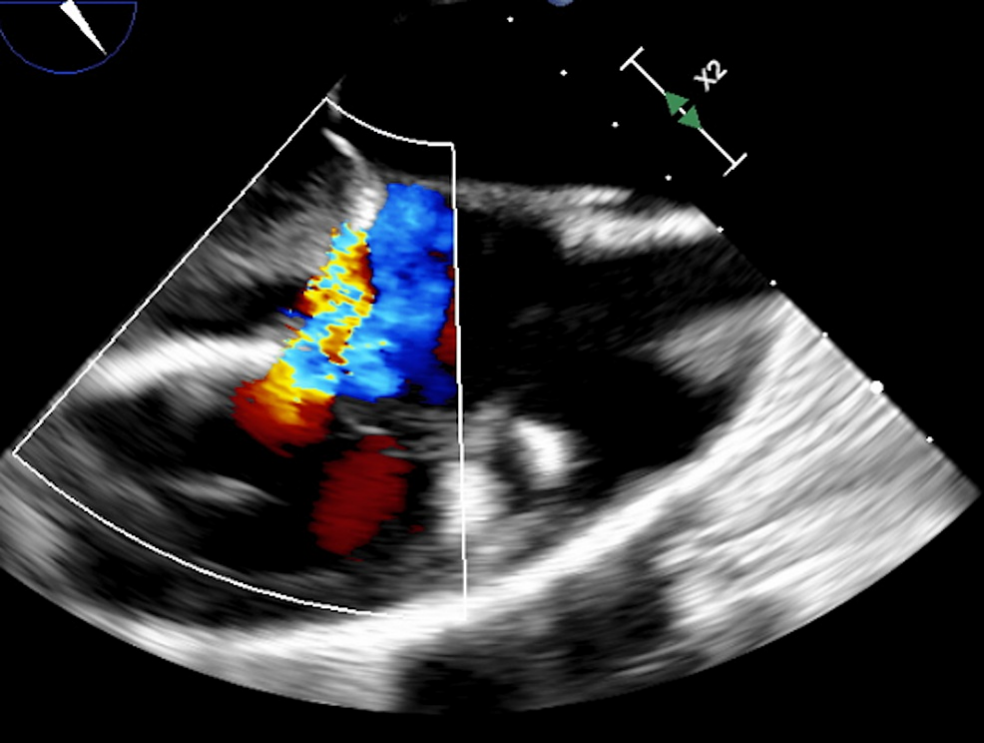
Severe tricuspid regurgitation noted on transesophageal echocardiogram.
